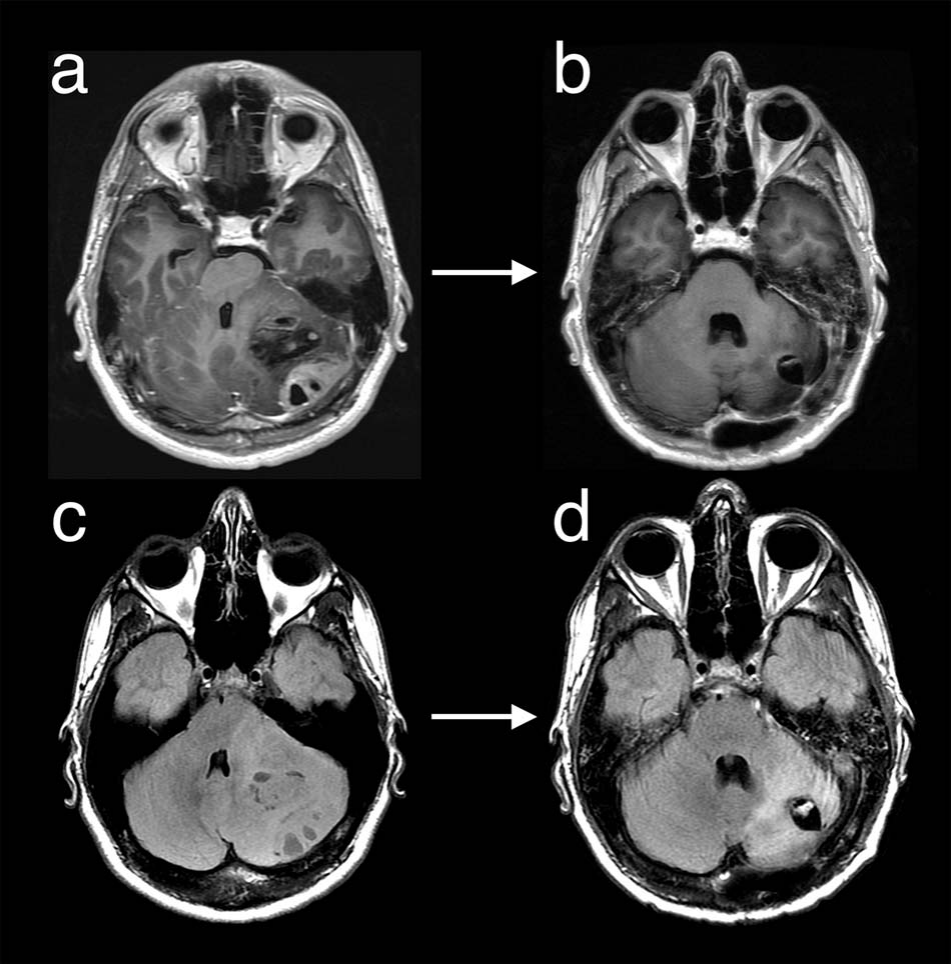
MRI with Axial T1 post-contrast (a/b) and Axial T2 FLAIR (c/d) demonstrate a large heterogeneously enhancing mass centred in the left cerebellar hemisphere with significant mass effect at diagnosis (a/c). After initial therapy with surgical debulking, ICE chemotherapy and cranio-spinal radiotherapy there was no residual enhancing disease (b) with significant reduction in non-enhancing T2 FLAIR signal (d).
